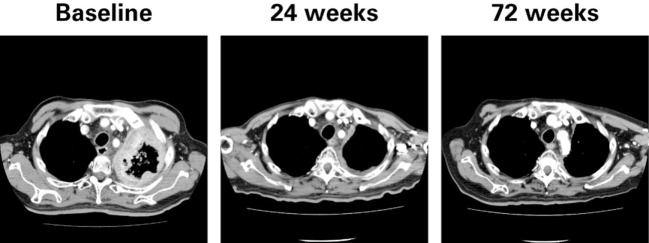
Computed tomography scans of a representative patient with anaplastic thyroid cancer at baseline and during treatment with lenvatinib.
